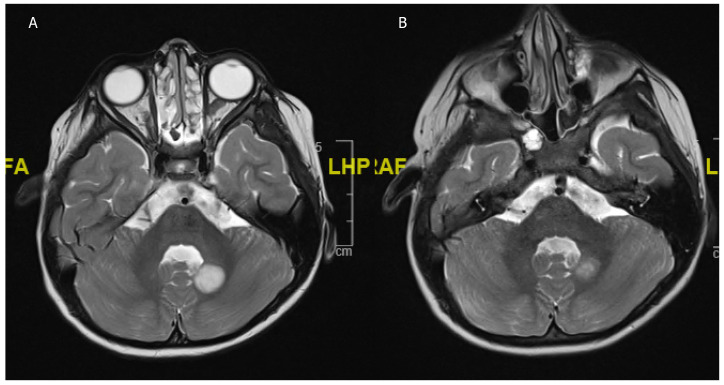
An 8 year-old girl undergoing treatment for congenital scoliosis. (A) MRI head carried out in the setting of her scoliosis work-up showed an incidental finding of a T2 hyperintense cerebellar lesion (B) Interval scan after 6 months showed a spontaneous regression of the lesion. No resection or biopsy was attempted.
